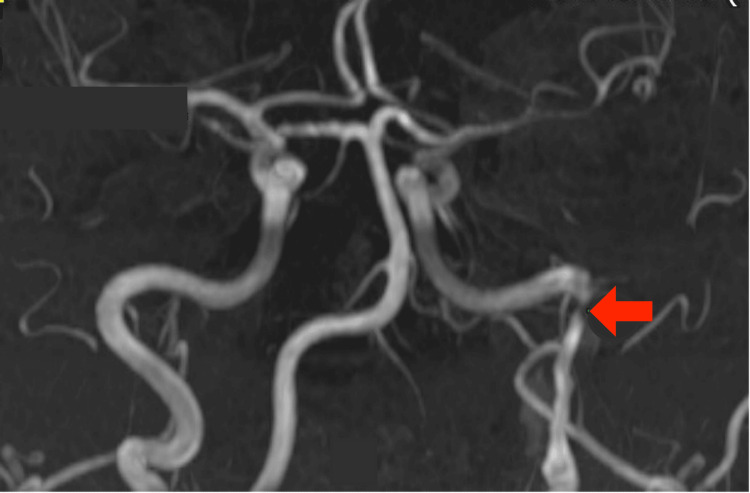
Reconstructed MRI angiography (bone window excluded) demonstrating a long-segment dissection with associated stenosis of the left ICA, extending from the cervical region to the skull base
